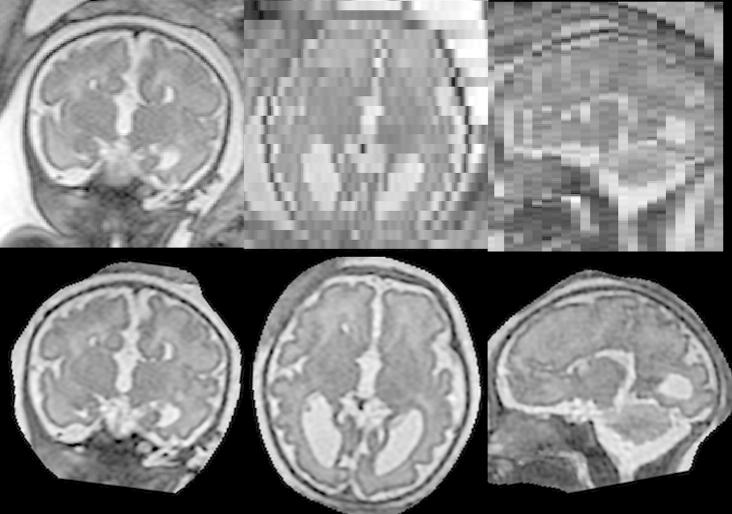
Reconstructed fetal brain MRI of a subject with GA 34 weeks using only five stacks of slices. First row: One of the acquired stacks. Second row: Reconstructed volume.
